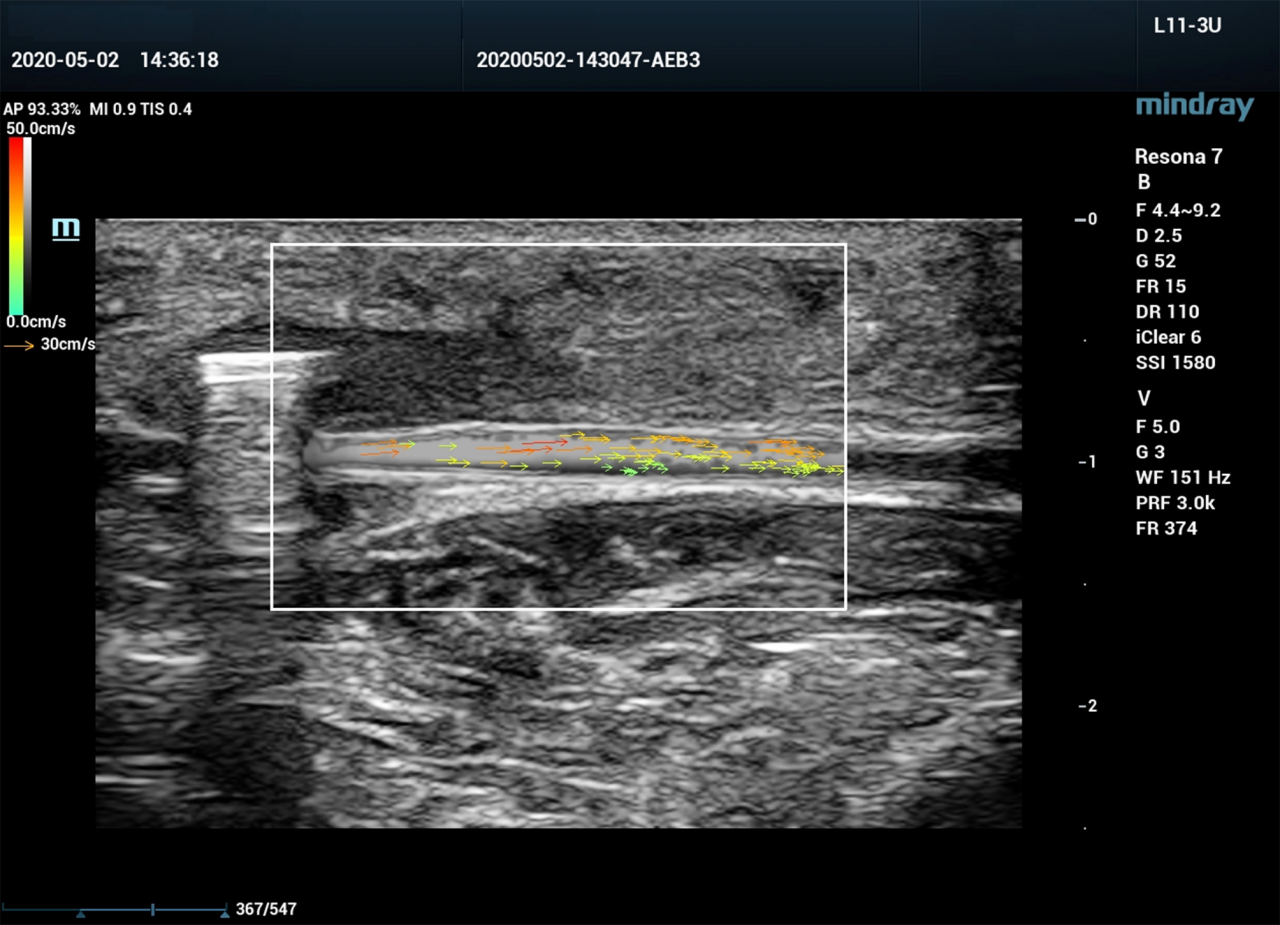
V Flow ultrasound image of a canine femoral artery with post-stenotic segment spasm.
